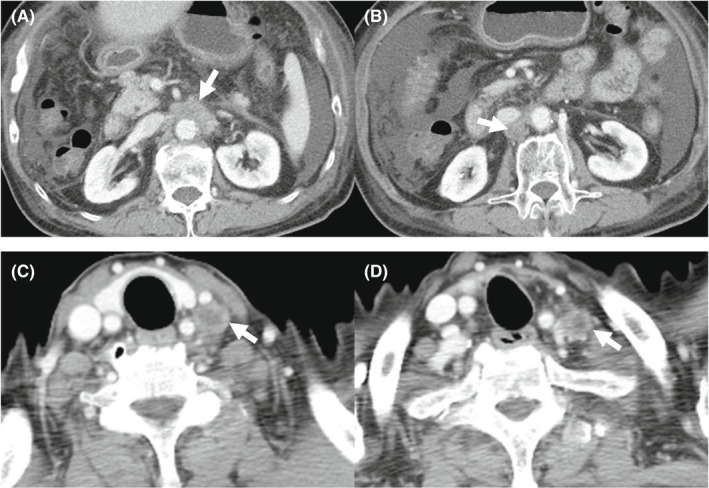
Enhanced computed tomography after two cycles of therapy showed mild shrinkage of enlarged lymph nodes, and the response rate per RECIST was determined to be stable disease. (A) Lymph nodes around the abdominal aorta. (B) A lymph node behind the inferior vena cava. (C, D) Left supraclavicular lymph nodes
